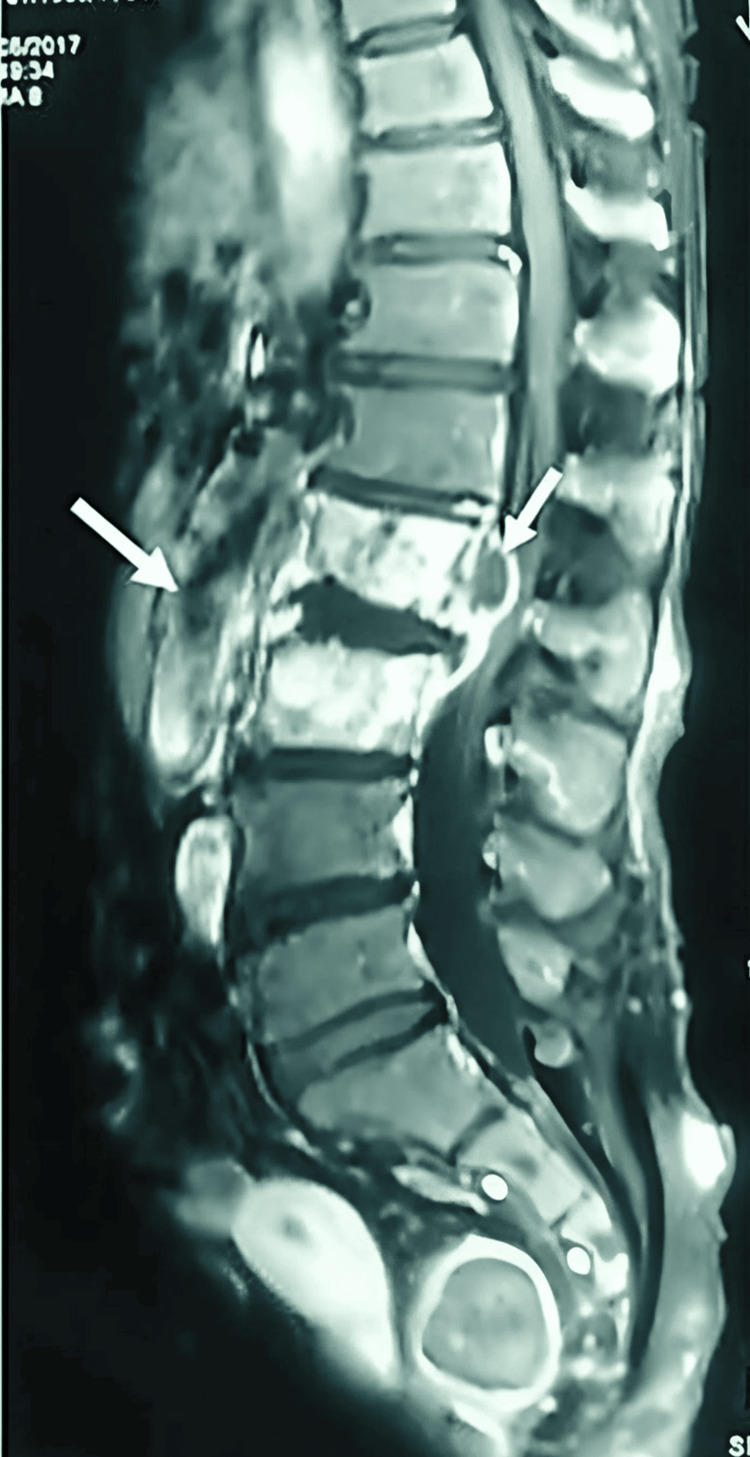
Sagittal view of a T2-weighted magnetic resonance imaging showing Short-Tau Inversion-Recovery (STIR) hyperintensity of the L2 and L3 vertebral bodies with highly enhancing STIR hyperintensity of the L1-L2 disc, raising the suspicion of contiguous discitis associated with anterior epidural abscesses extending over 4 cm
